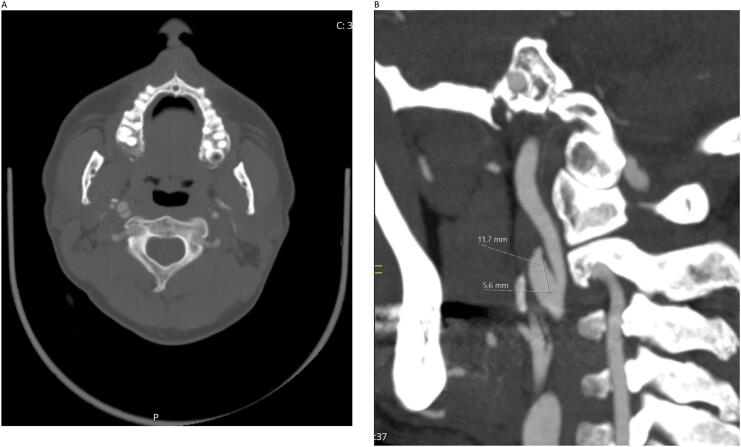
A: CT angiography coronal cut showing normally appearing left ICA with no dissection, with right ICA dissection. B: Axial cut. C: Smaller 11.7 mm right ICA pseudoaneurysm.
